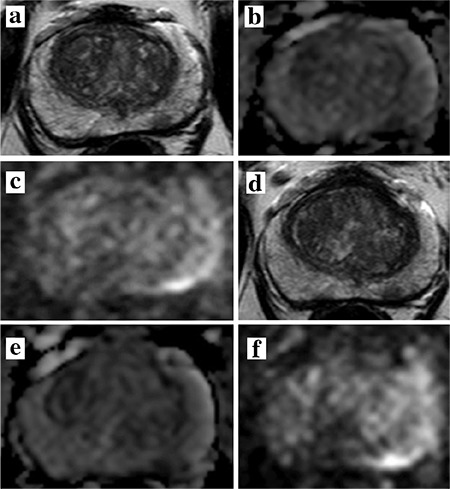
Sixty-year old man with a serum PSA= 4.58 ng/mL. Baseline mpMRI consisted of T2W MRI (a) ADC map (b) b1500 DW MRI (c) shows a focal lesion in the left mid peripheral zone. TRUS/MRI fusion guided biopsy revealed Gleason 3+3 prostate cancer within the lesion. One year follow up axial T2W MRI (d) ADC map (e) and b1500 DW MRI (f) shows no significant change within the lesion (serum PSA at 1 year follow up= 5.10 ng/mL). TRUS/MRI fusion biopsy revealed Gleason 3+3 with this lesion and patient continues to remain on active surveillance.
